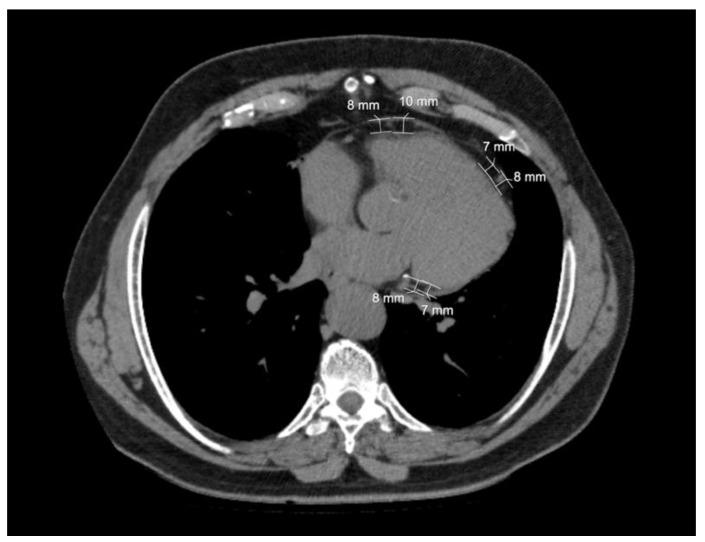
Measurement of epicardial adipose tissue thickness on axial thoracic computed tomography images. Special attention should be paid to ensuring that the measurement regions correspond specifically to perivascular adipose tissue.
